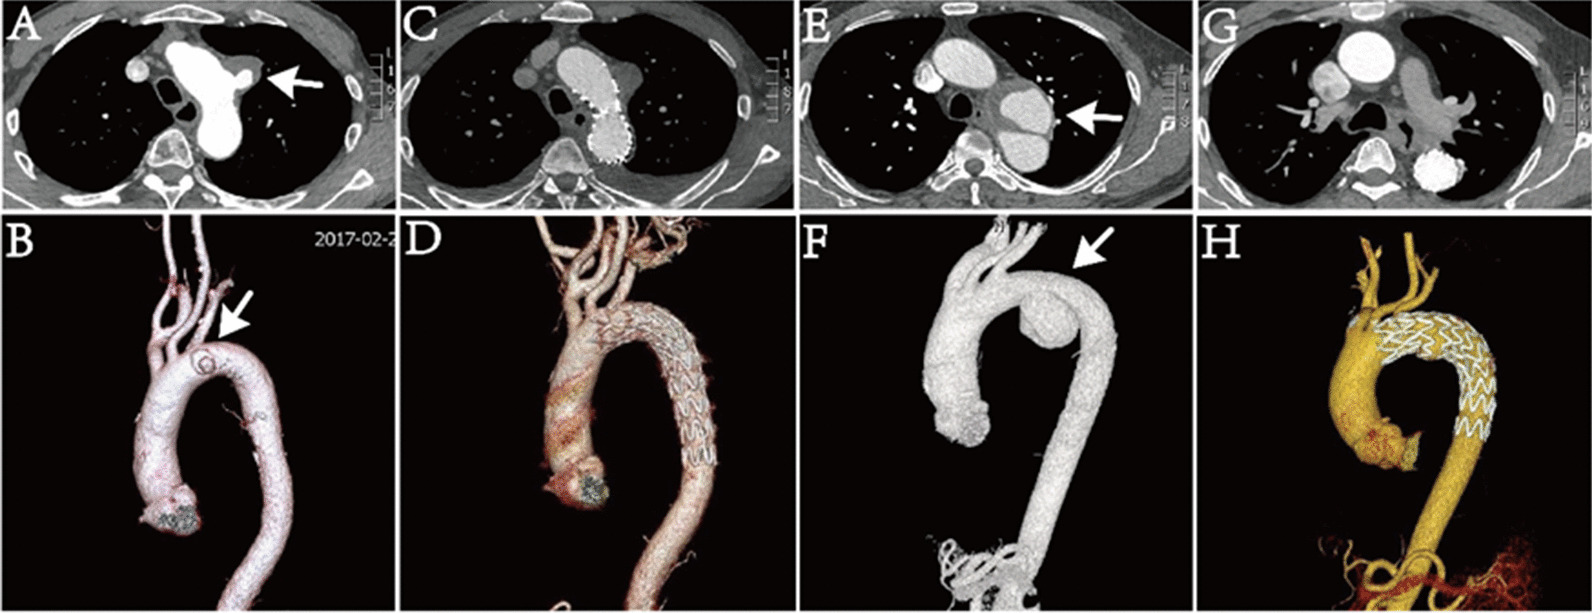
A–D Preoperative and 1-week postoperative CTA images of a thoracic TAP patient receiving TEVAR and in vitro fenestration of LSA. E–H Preoperative and 5-year postoperative CTA images of a thoracic TAP patient undergoing TEVAR
